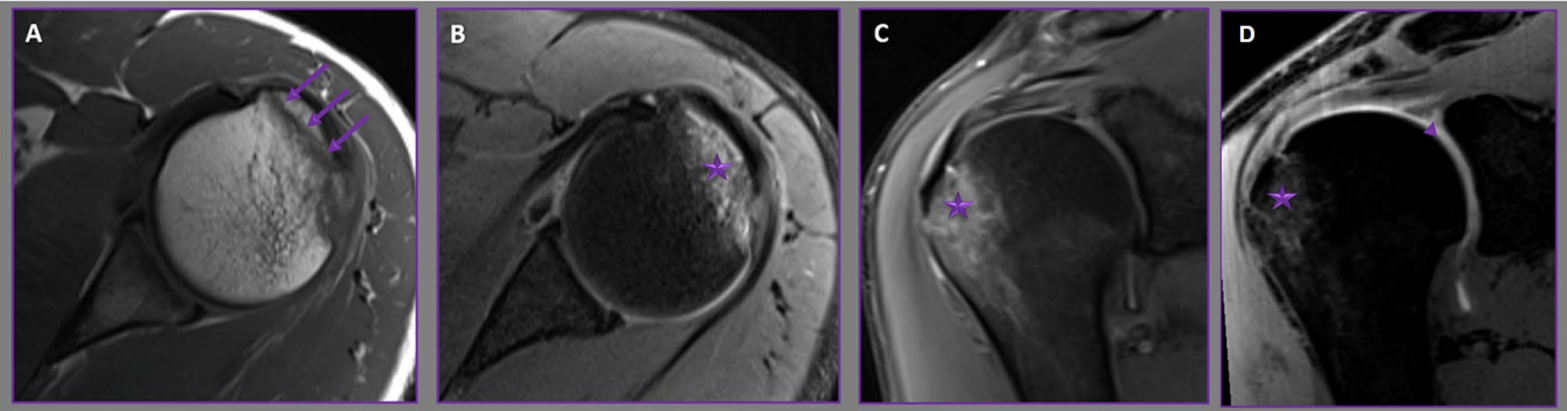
Axial T1 (A)- and PD-weighted(B) and oblique coronal PD-weighted (C) MR images demonstrate greater tuberosity avulsion fracture (arrows) and its associated bone marrow edema (stars). Oblique coronal fat-suppressed T1-weighted VIBE MR arthrogram (D) shows greater tuberosity avulsion fracture (star) and its associated SLAP lesion (arrow head)
